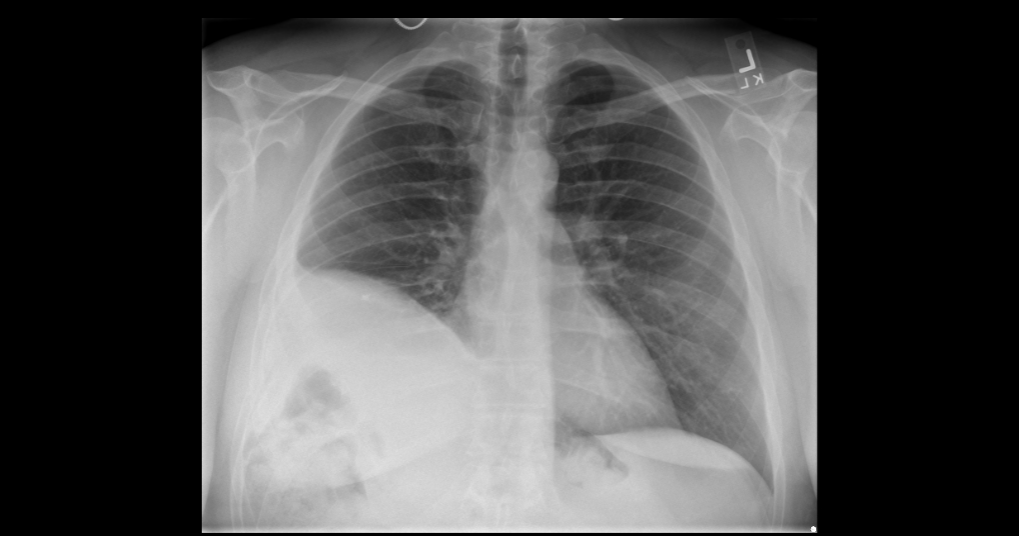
Chest X-ray following surgery. Posteroanterior view of his chest one year after repair. His diaphragm is elevated due to the use of prosthetic mesh and the repair is intact without evidence of recurrence of the hernia.
